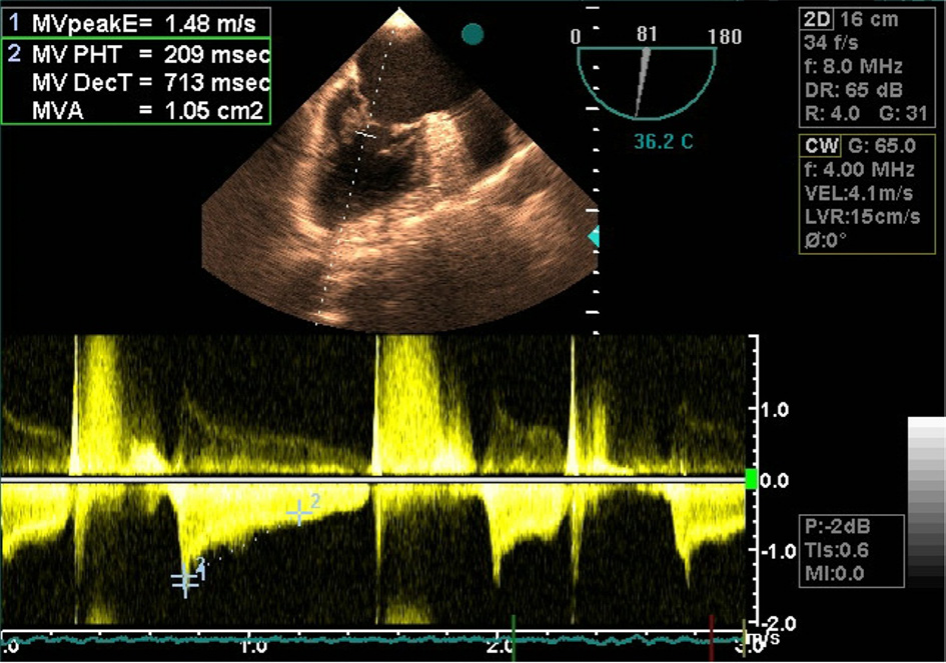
Transesophageal echo before surgery
